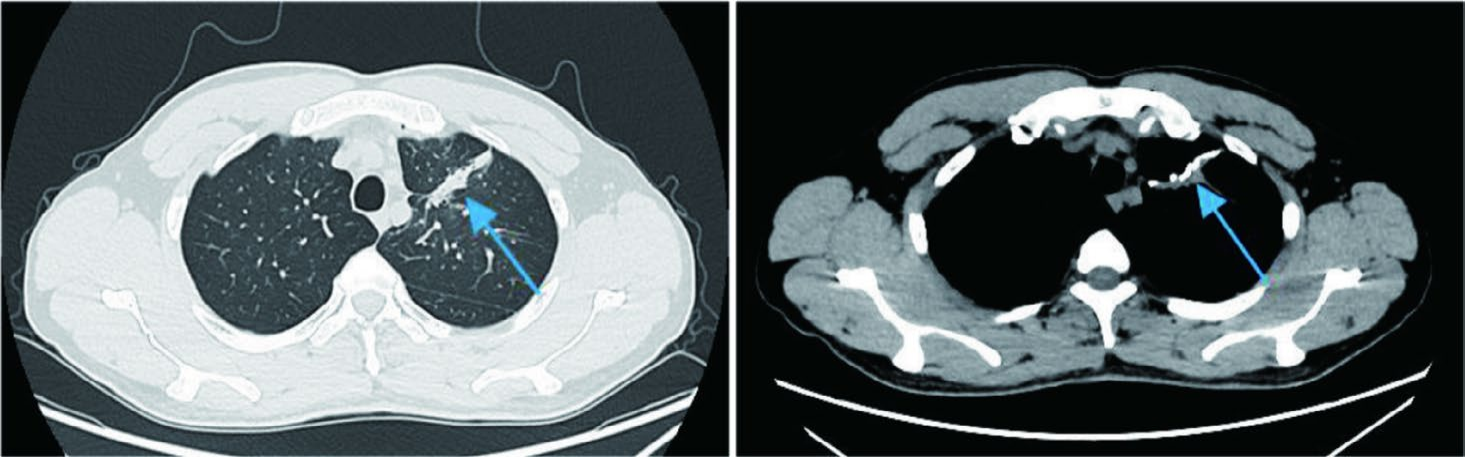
The nodule is close to the periphery, so wedge resection is chosen during the operation. After the operation, the CT scan shows the shadow of the stapler chain. The tumor is resected with R0 resection while maximizing the preservation of lung function. The lung expands completely after the operation, and the postoperative recovery is good.
